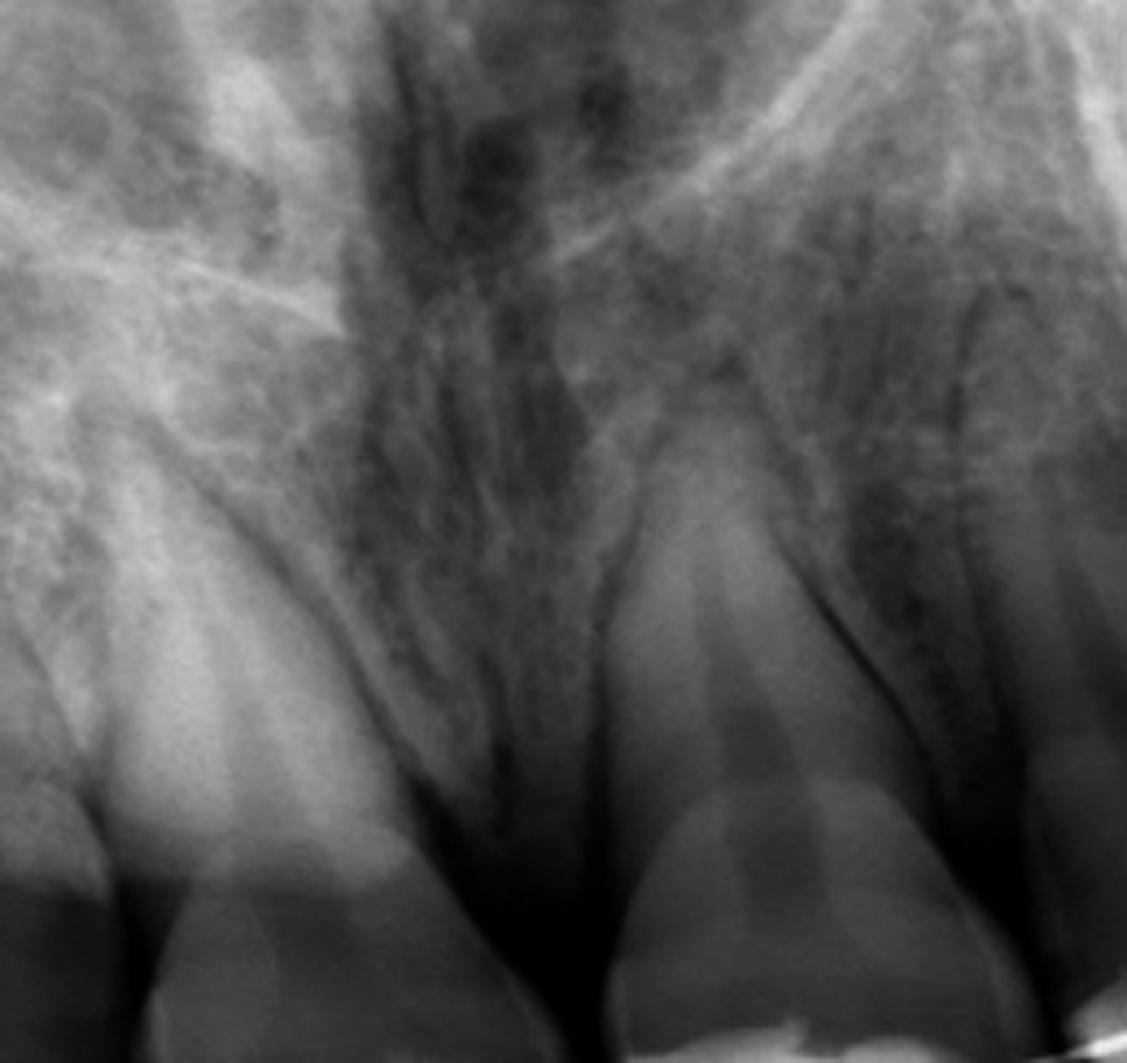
Periapical radiography after reduction
